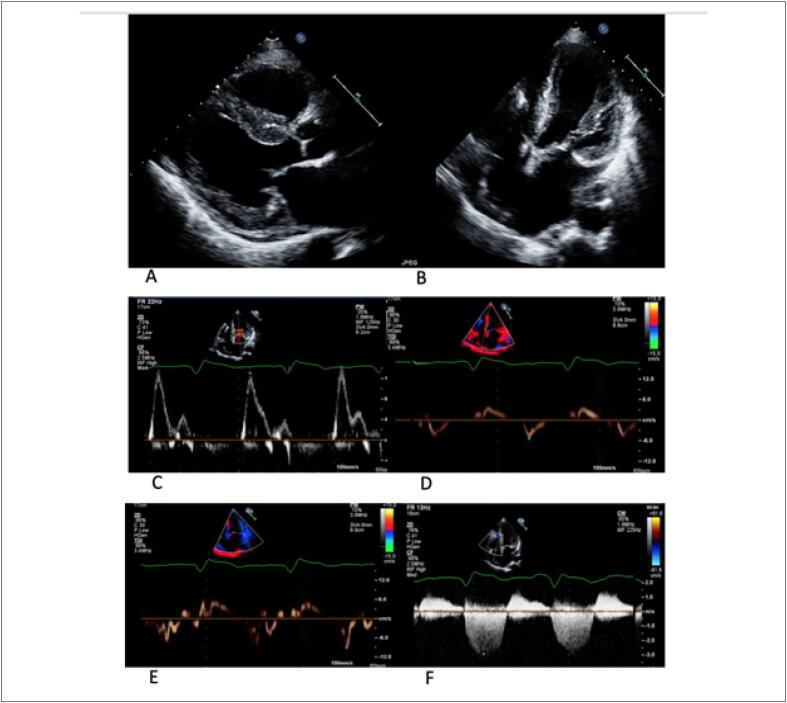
Achados da ecocardiografia transtorácica. Os painéis A (projeção paraesternal de eixo longo) e B (apical de 4 câmaras) mostram aumento da espessura da parede (máximo de 15 mm no septo interventricular basal) e átrio esquerdo levemente dilatado (volume indexado pela superfície corporal de 40 mL / m^2^). O painel C mostra uma razão E/A aumentada de 3,4. O painel D mostra uma velocidade de e' lateral de 9 cm/s e o painel E uma e' septal de 5 cm/s, dando uma média E/e' de 15. No painel F, a velocidade de regurgitação tricúspide é estimada em 2,9 m/s com Cw Doppler. Portanto, a paciente preenchia os critérios para disfunção diastólica.
